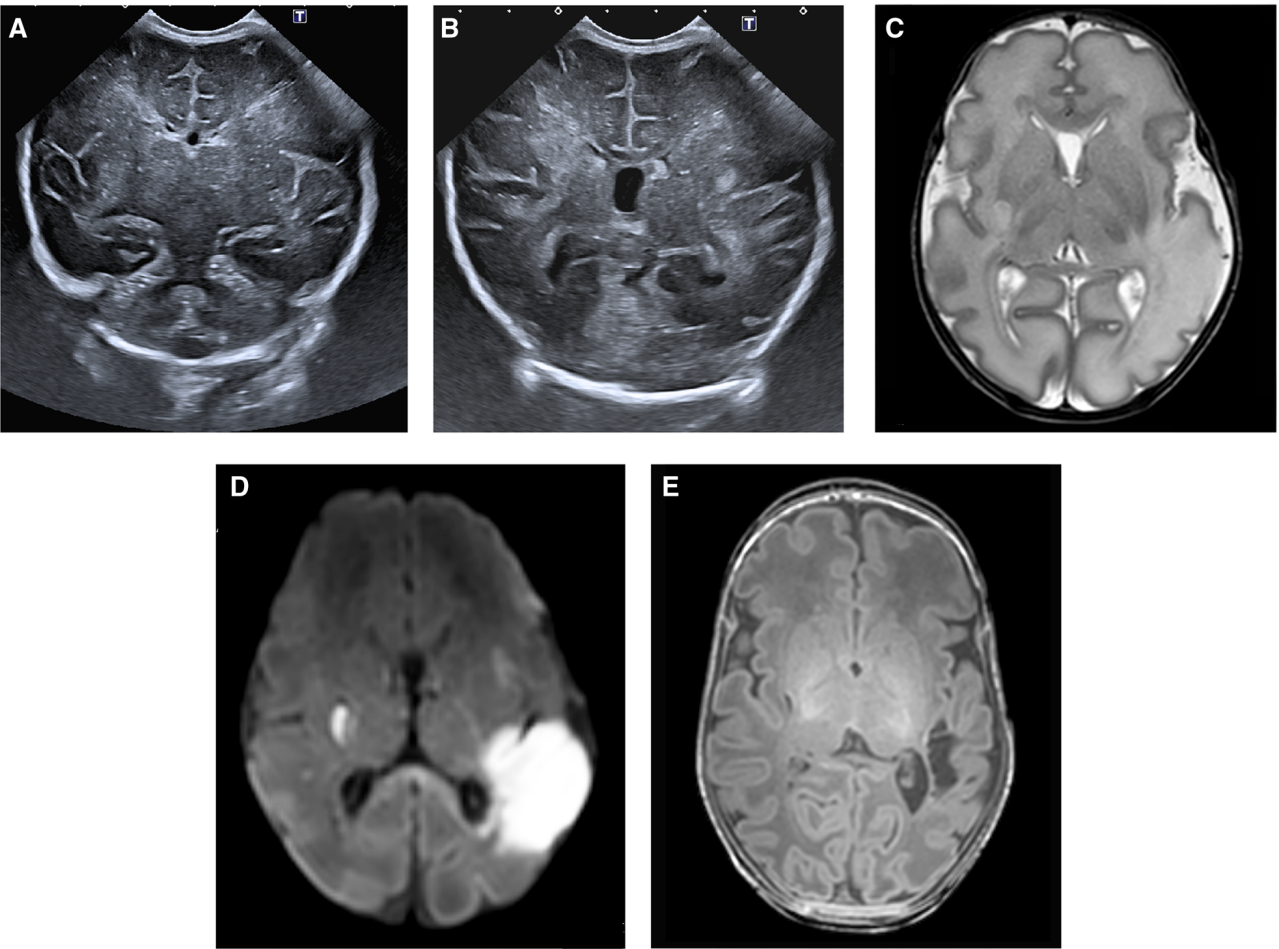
Case of direct perinatal arterial ischemic stroke, involving posterior middle cerebral artery (MCA) stroke left and perforator stroke right, in an ex-recipient twin complicated by twin-to-twin transfusion syndrome (Q1) and treated with complete fetoscopic laser surgery (twin 11). A, Cranial ultrasound (cUS) in coronal plane on postnatal day 4, revealing a small focus of increased echogenicity in the posterior right basal ganglia (encircled). B, cUS on the same day demonstrating bilateral white matter echogenicity, more pronounced on the left, extending towards the cortex. C, An MCA stroke was suspected, and therefore, a magnetic resonance imaging (MRI) on postnatal day 5 was indicated, showing on the axial T2-weighted image high signal intensity in the territory of the left posterior MCA branch, extending to the cortex, as well as the posterior right basal ganglia adjacent to the posterior limb of the internal capsule (PLIC). D, Diffusion-weighted imaging on the same day, showing diffusion restriction in both regions, confirming an acute stroke. E, A T1-weighted MRI at term-equivalent age demonstrating tissue loss in the posterior right basal ganglia and a small cyst in the right putamen and asymmetrical myelination of the PLIC, better on the left than right. The left-sided lesion in region of the posterior branch of the MCA had evolved into a cystic lesion adjacent to the left optic radiation, with white matter loss but relative preservation of the cortex. Clinically, the child developed early asymmetry of motor function, ultimately developing unilateral spastic cerebral palsy on the left (level 1). In addition, there is a risk of visual field asymmetry and later epilepsy (Table S3—twin 11).
